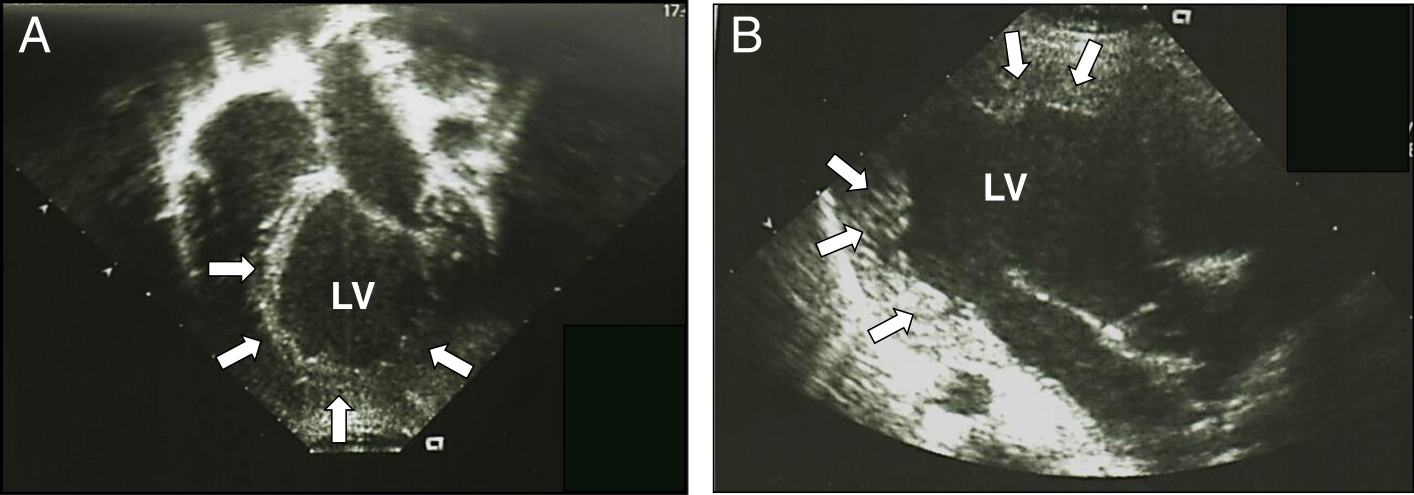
Echocardiographic images of the index patient. Both in apical four chamber (A) and in parasternal long axis view (B). The left ventricle (LV) appears dilated and spherical in shape. Note the preserved thickness of the parietal and septal myocardium with the relative prominence of the trabeculae of the apex and lateral wall (arrows).
